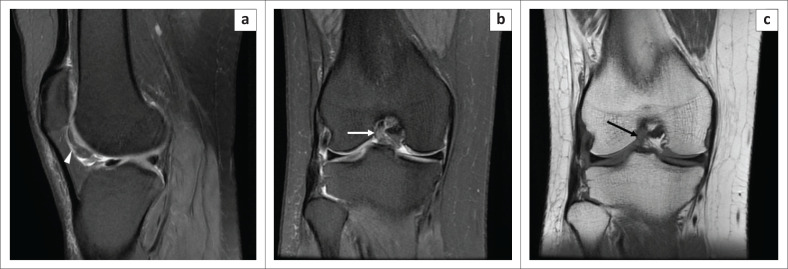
Magnetic resonance imaging of an infrapatellar plica tear in a 33-year-old patient with a twisting injury to the knee and clinically-suspected anterior cruciate ligament (ACL) injury: oblique sagittal PDFS image (a) revealed a hyperintense fluid collection along the entire course of the infrapatellar plica (arrow head), with irregular margins. Coronal PDFS image shows hyperintense signal along the ACL with intact fibres suggestive of mild sprain in the postero-lateral bundle of the ACL (arrow). Corresponding signal alteration on T1WI is shown in (c-arrow).
